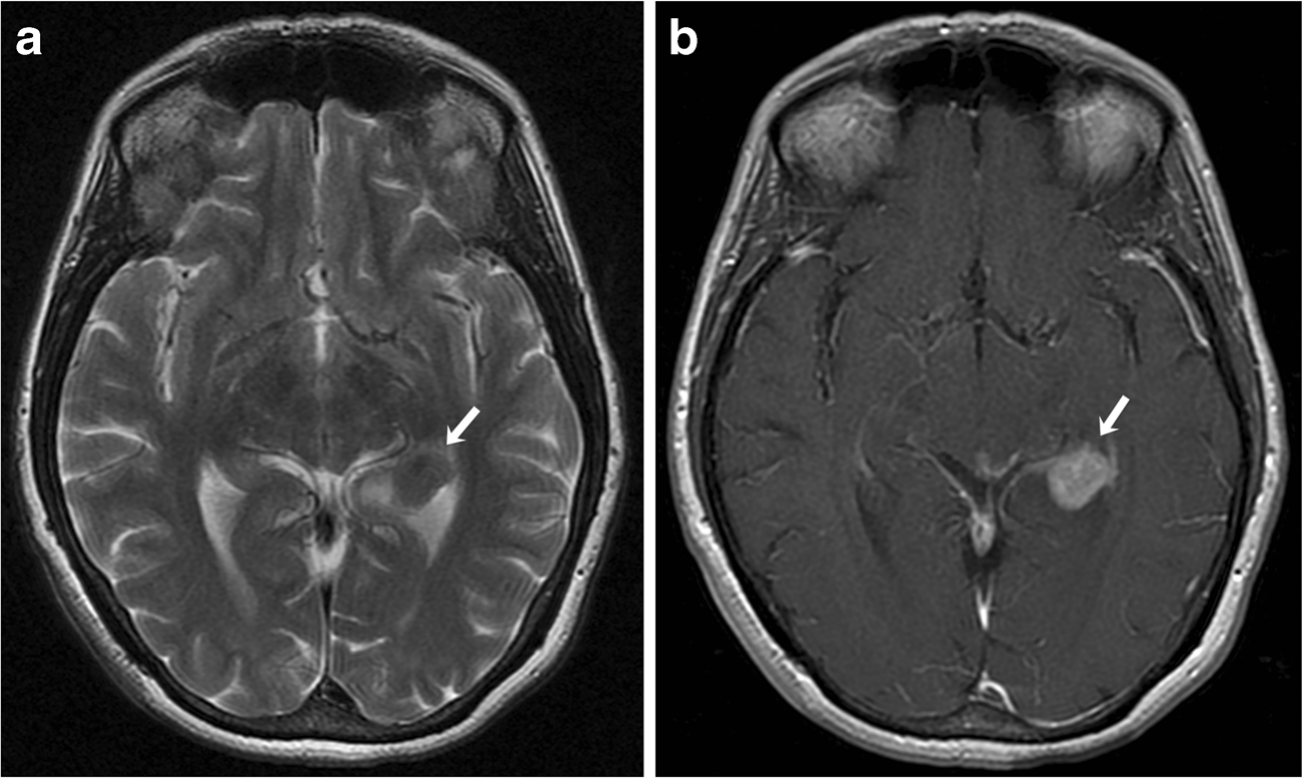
A 54-year-old female with choroidal melanoma. a Axial T2-weighted MR image showing an intraventricular mass lesion with T2 shortening involving the left lateral ventricle (arrow). b On the corresponding axial contrast-enhanced T1-weighted MR image, the lesion shows avid enhancement (arrow)
